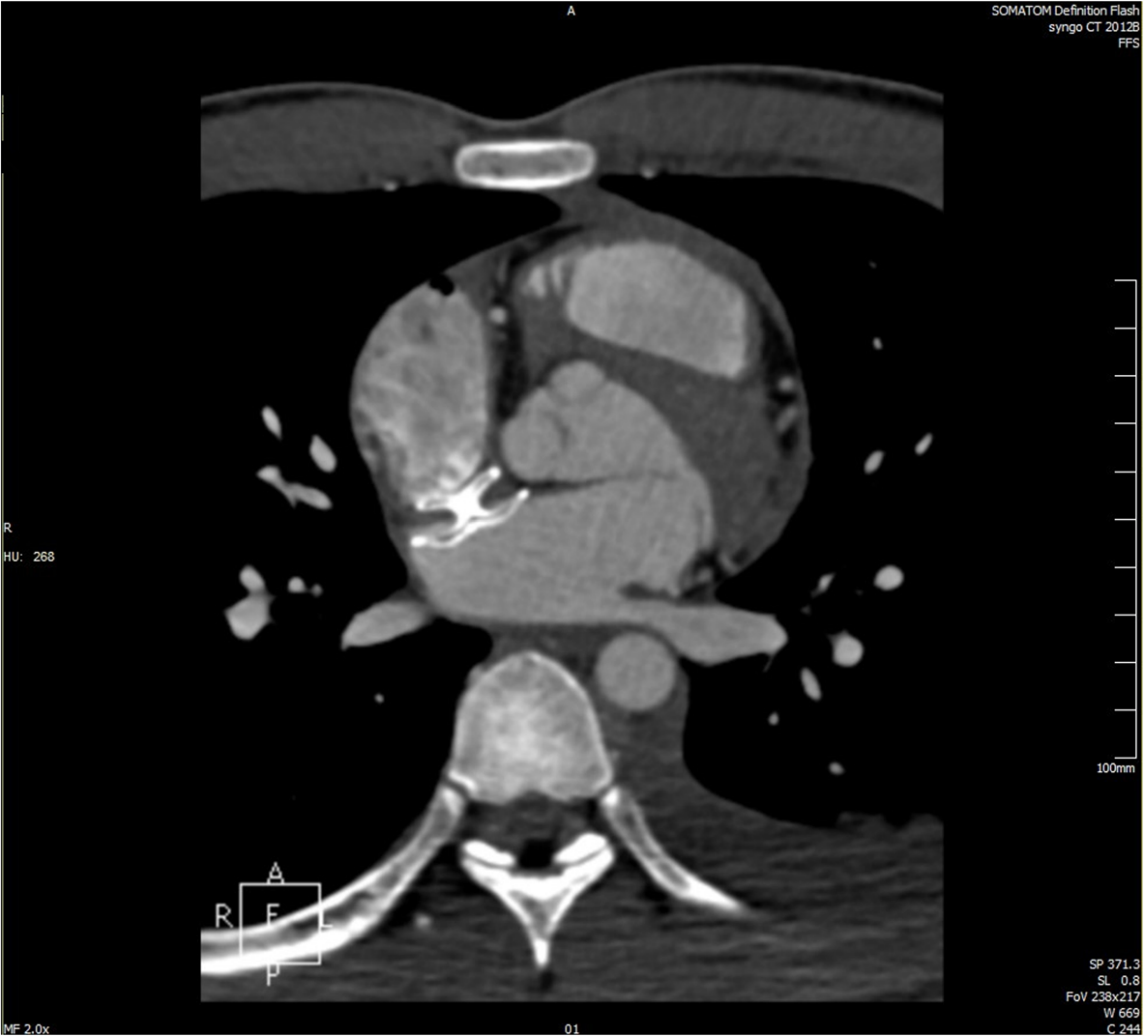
After the patient developed chest pain, cardiac CT showed changes in the atrial septal defect since transcatheter closure and moderate pericardial effusion
